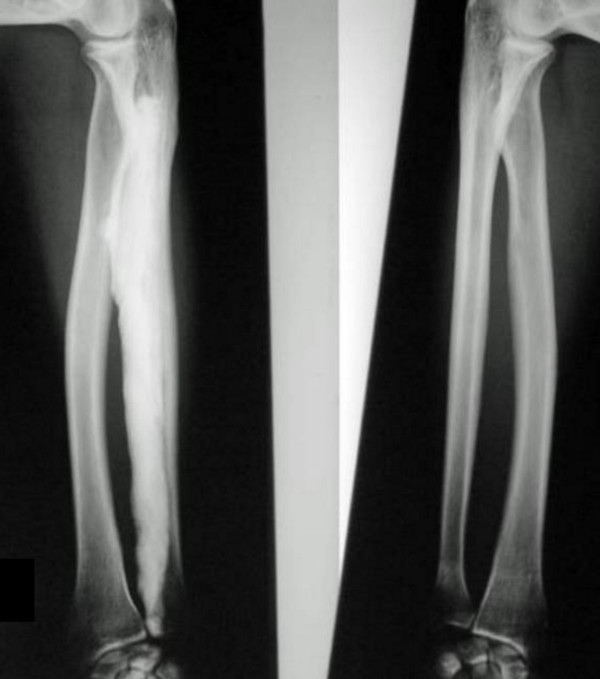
Radiograph of the forearms showed, typical cortical thickening, mostly along the right radius, massive extensive thickening and sclerosis extends along the carpal bones respectively. There was mild sclerosis of the ulnae with bowing; similarly the radius on the left side is also mildly sclerotic.
